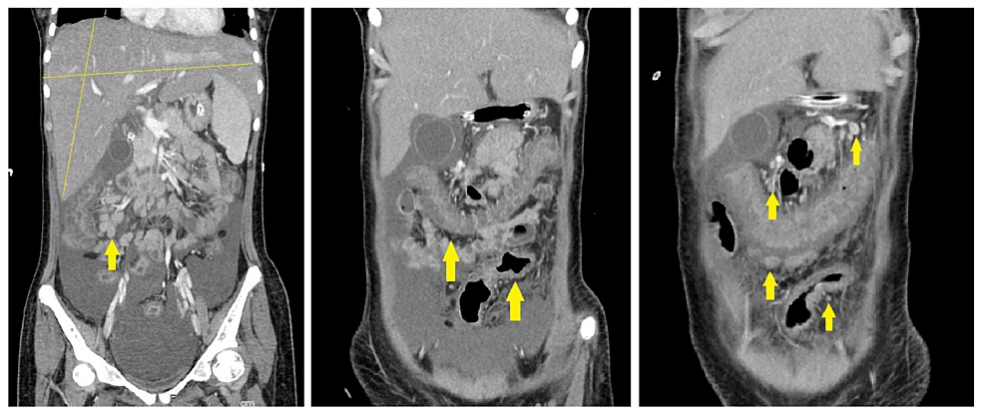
Multiple CT scan planes of abdomen and pelvis showing hepatosplenomegaly, moderate ascites, and multiple enlarged lymph nodes. CT: computed tomography.
